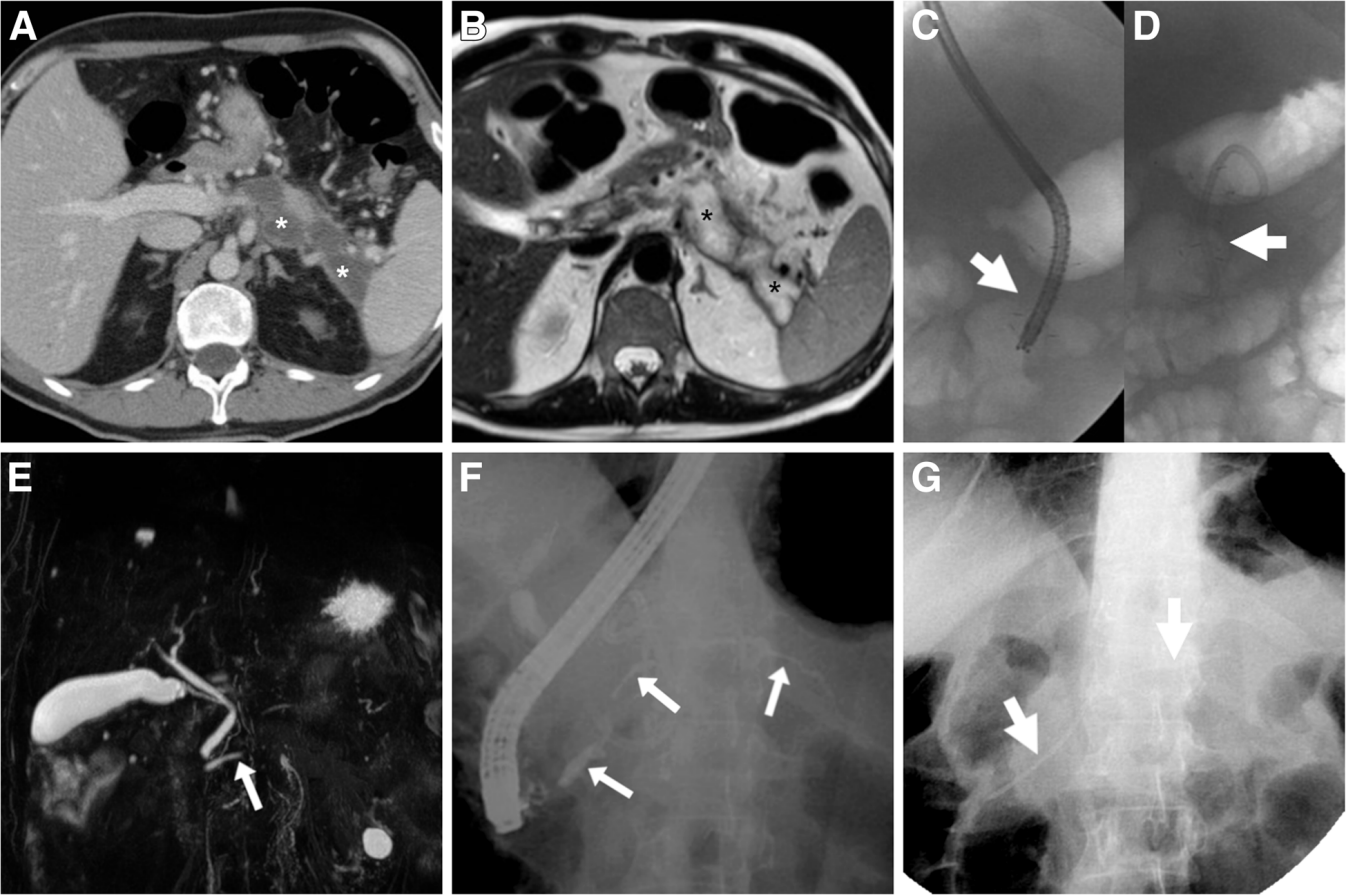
In a 56-year-old male, a year after severe necrotic-haemorrhagic AP, T2-weighted MRI (a) and contrast-enhanced MDCT (b) showed persistent ample regions of hyperintense, nonenhancing parenchymal necrosis (*). At another hospital, endoscopic ultrasound (EUS)-guided cystogastrostomy was initially performed, including positioning of a 3-cm-long, 12-mm-wide self-expanding metal stent (thick arrow in c). After obtaining incomplete regression of necrotic collection, repeated endoscopy at our institution included trans-stent necrosectomy and deployment of a plastic pigtail stent (thick arrow in d). After poor, prolonged clinical improvement, MRCP (e) showed mild MPD dilatation at the pancreatic head (arrow) and segmental discontinuity at the body. Diagnosis of disconnected pancreatic duct syndrome (DPDS) was confirmed during endoscopic retrograde cholangiopancreatography (ERCP, f) and definitively treated by positioning of a long stent (thick arrows in g) through the disrupted MPD, ultimately resulting in relieved complaints and laboratory changes (adapted from Open Access ref. no. [27])
